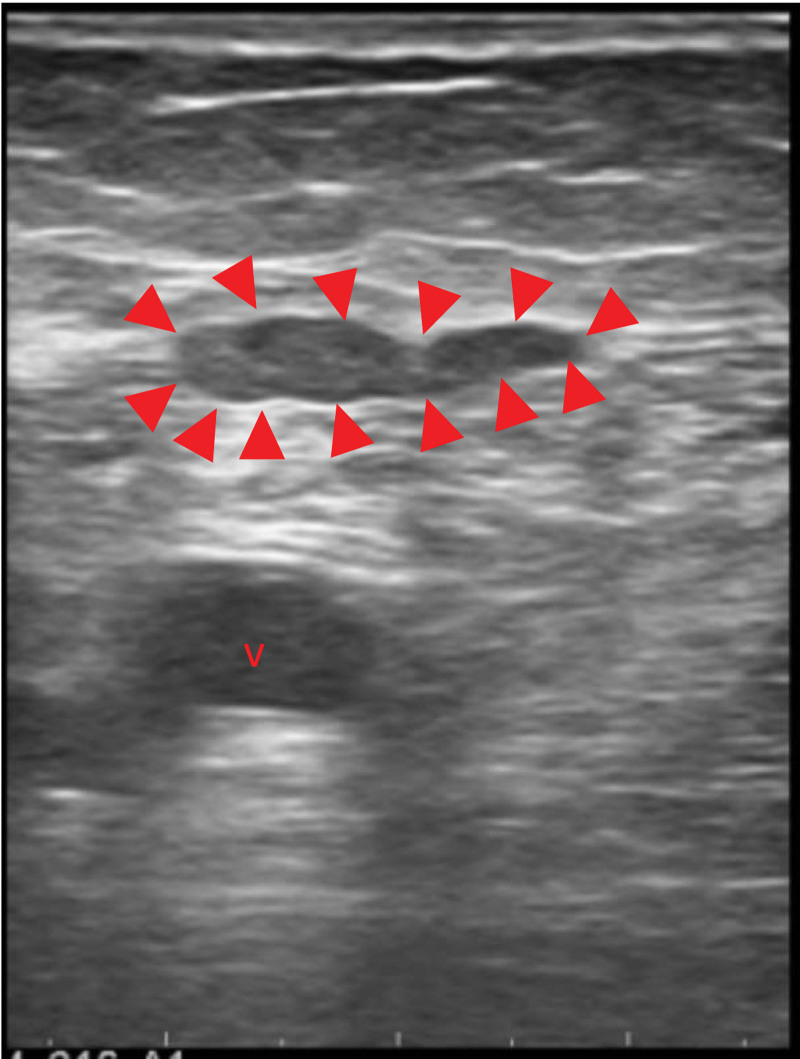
The intraoperative sonography during the first autograftectomy showed only the larger parathyroid mass (1.7 7cm × 0.37cm, arrow head) and was removed, but the smaller one was not definitely identified under sonography during operation and was left behind with the idea that even if the small remnant tissue not being removed might not be harmful afterwards. (v: vessel).
